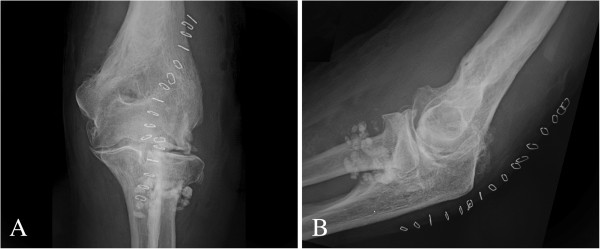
Anteroposterior (A) and lateral (B) postoperative radiographs of the left elbow.
